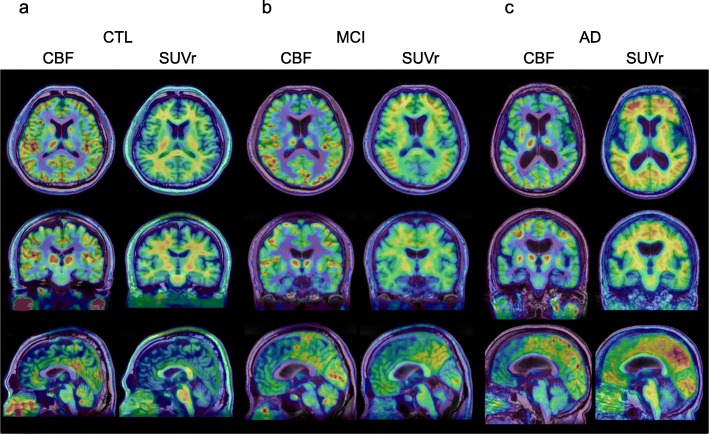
Representative CBF (left) and SUVr (right) images of healthy control (CTL) (a), MCI (b), and early AD (c) calculated from [^11^C]PiB-PET. MMSE scores were 30, 29, 25, respectively. SUVr images showed a gradual increase of cortical [^11^C]PiB accumulation according to severity of the disease, but CBF values were close between controls and MCI patients. CBF (mL/min/100 g) images showed similar distribution between MCI and CTL, while early AD patients showed slight decrease in frontal and temporal lobes. Note the posterior cingulate cortex and the precuneus did not show CBF decrease and gradual SUVr increase in the AD patient
